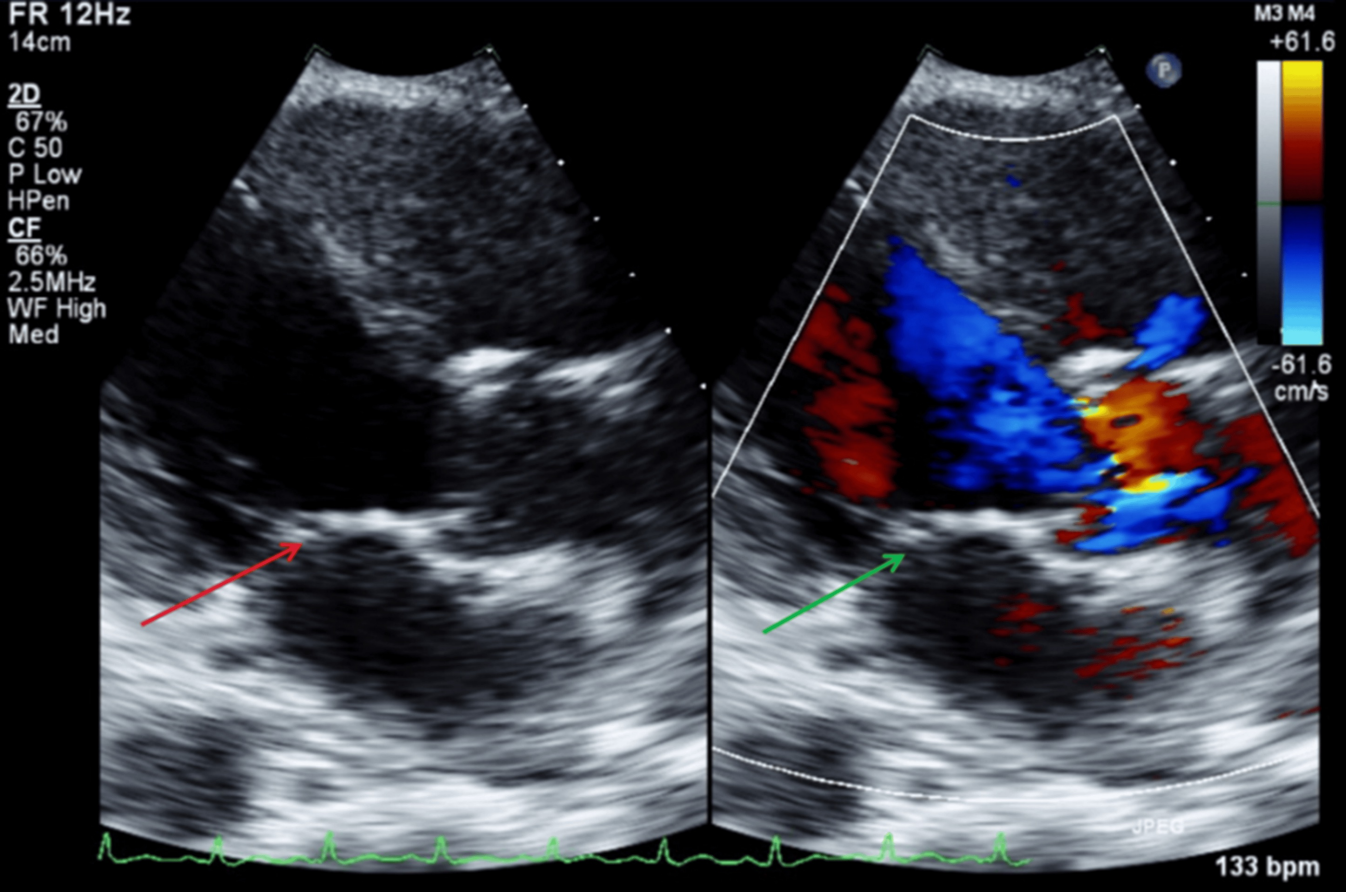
TTE parasternal long-axis view with and without color Doppler during systole. The red arrow shows mitral valve with no visible vegetation. The green arrow shows no findings of mitral valve regurgitation with color doppler. TTE: transthoracic echocardiography
